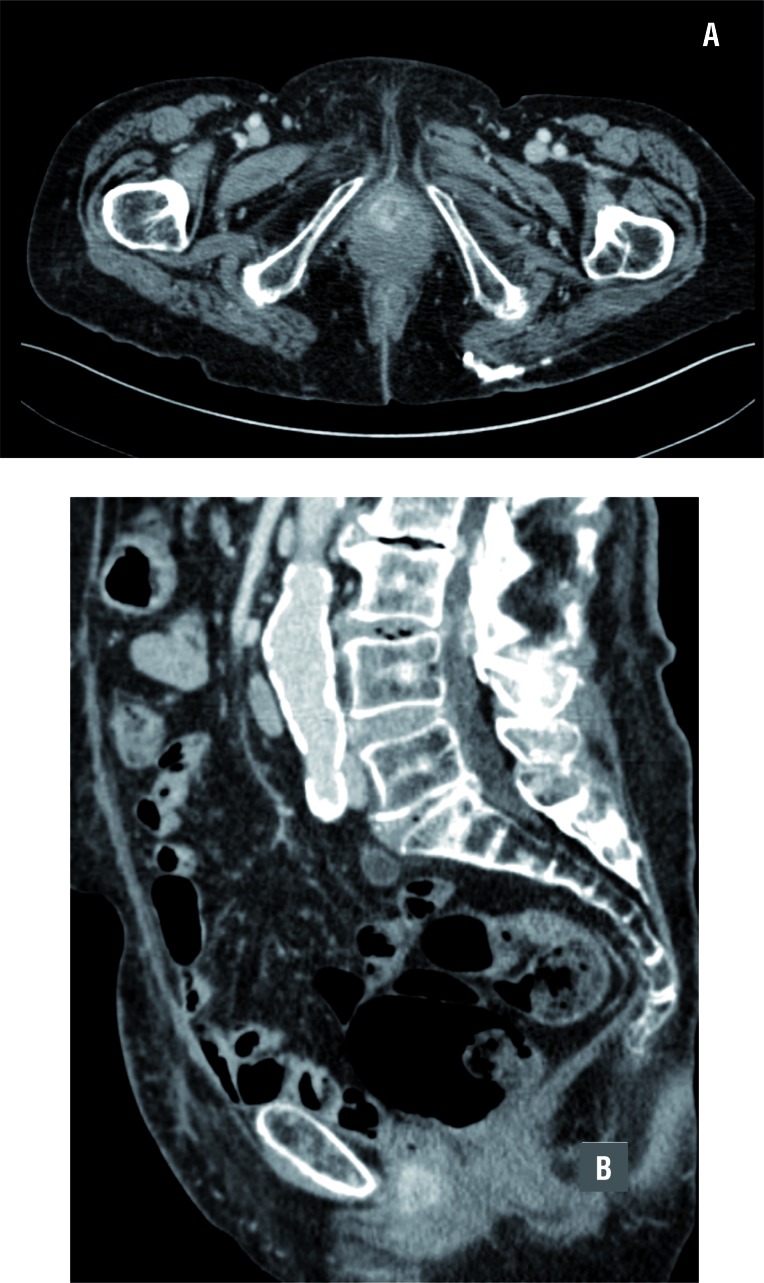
Vaginal cuff recurrence in an 82 year old female. After undergoing radical cystectomy and lymphadenectomy (pT3aN0 tumor), patient subsequently developed symptomatic vaginal blood spotting. The CT scan demonstrates a solid mass originating from the vaginal cuff. Surgery was performed and final pathology showed a recurrence of urothelial carcinoma originating from the vaginal cuff.
